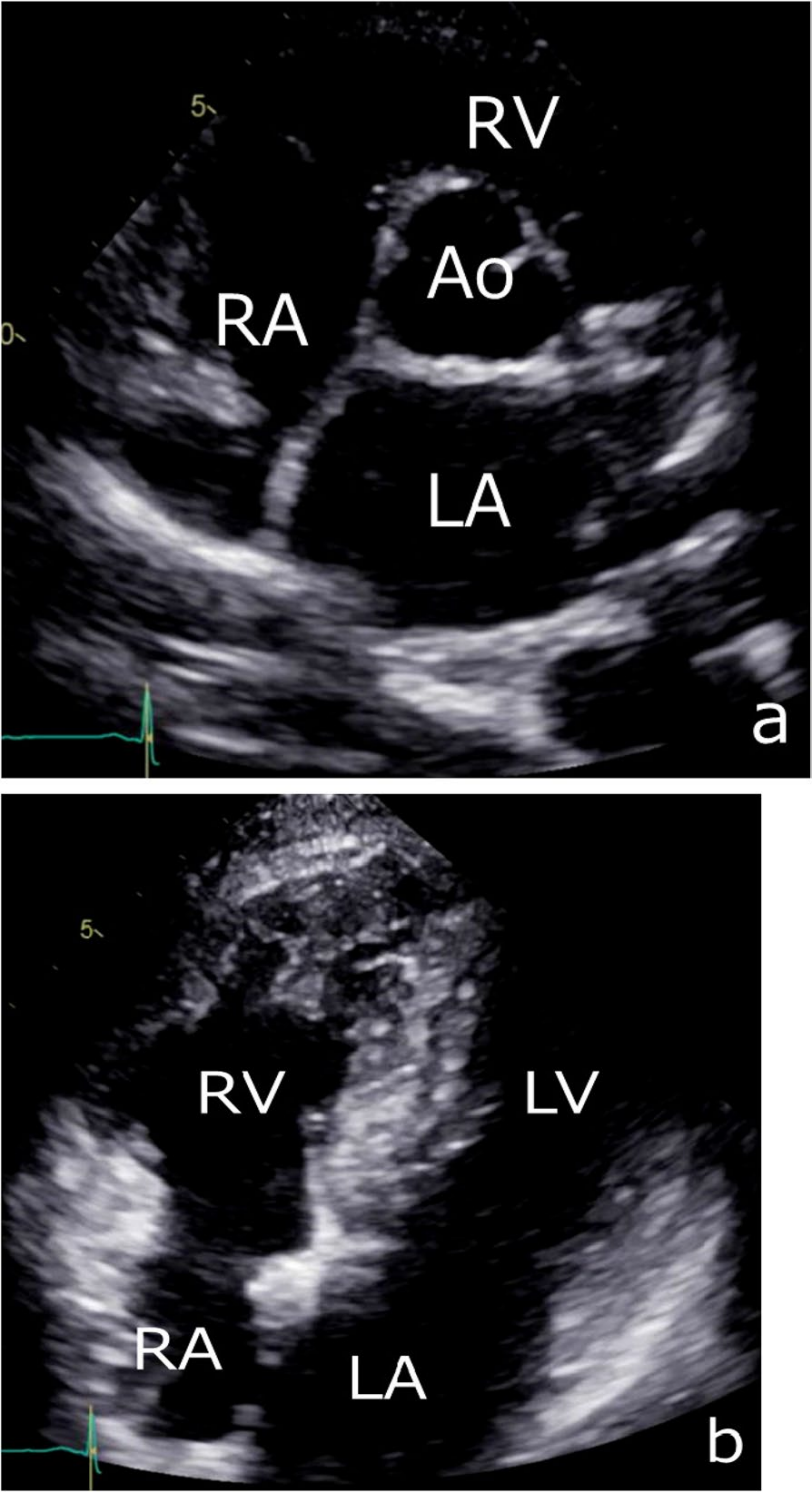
Postoperative transthoracic echocardiography showed RA volume reduction without transarterial shunt flow or residual mass. AV, aortic valve; LA, left atrium; LV, left ventricle; RA, right atrium; RV, right ventricle
